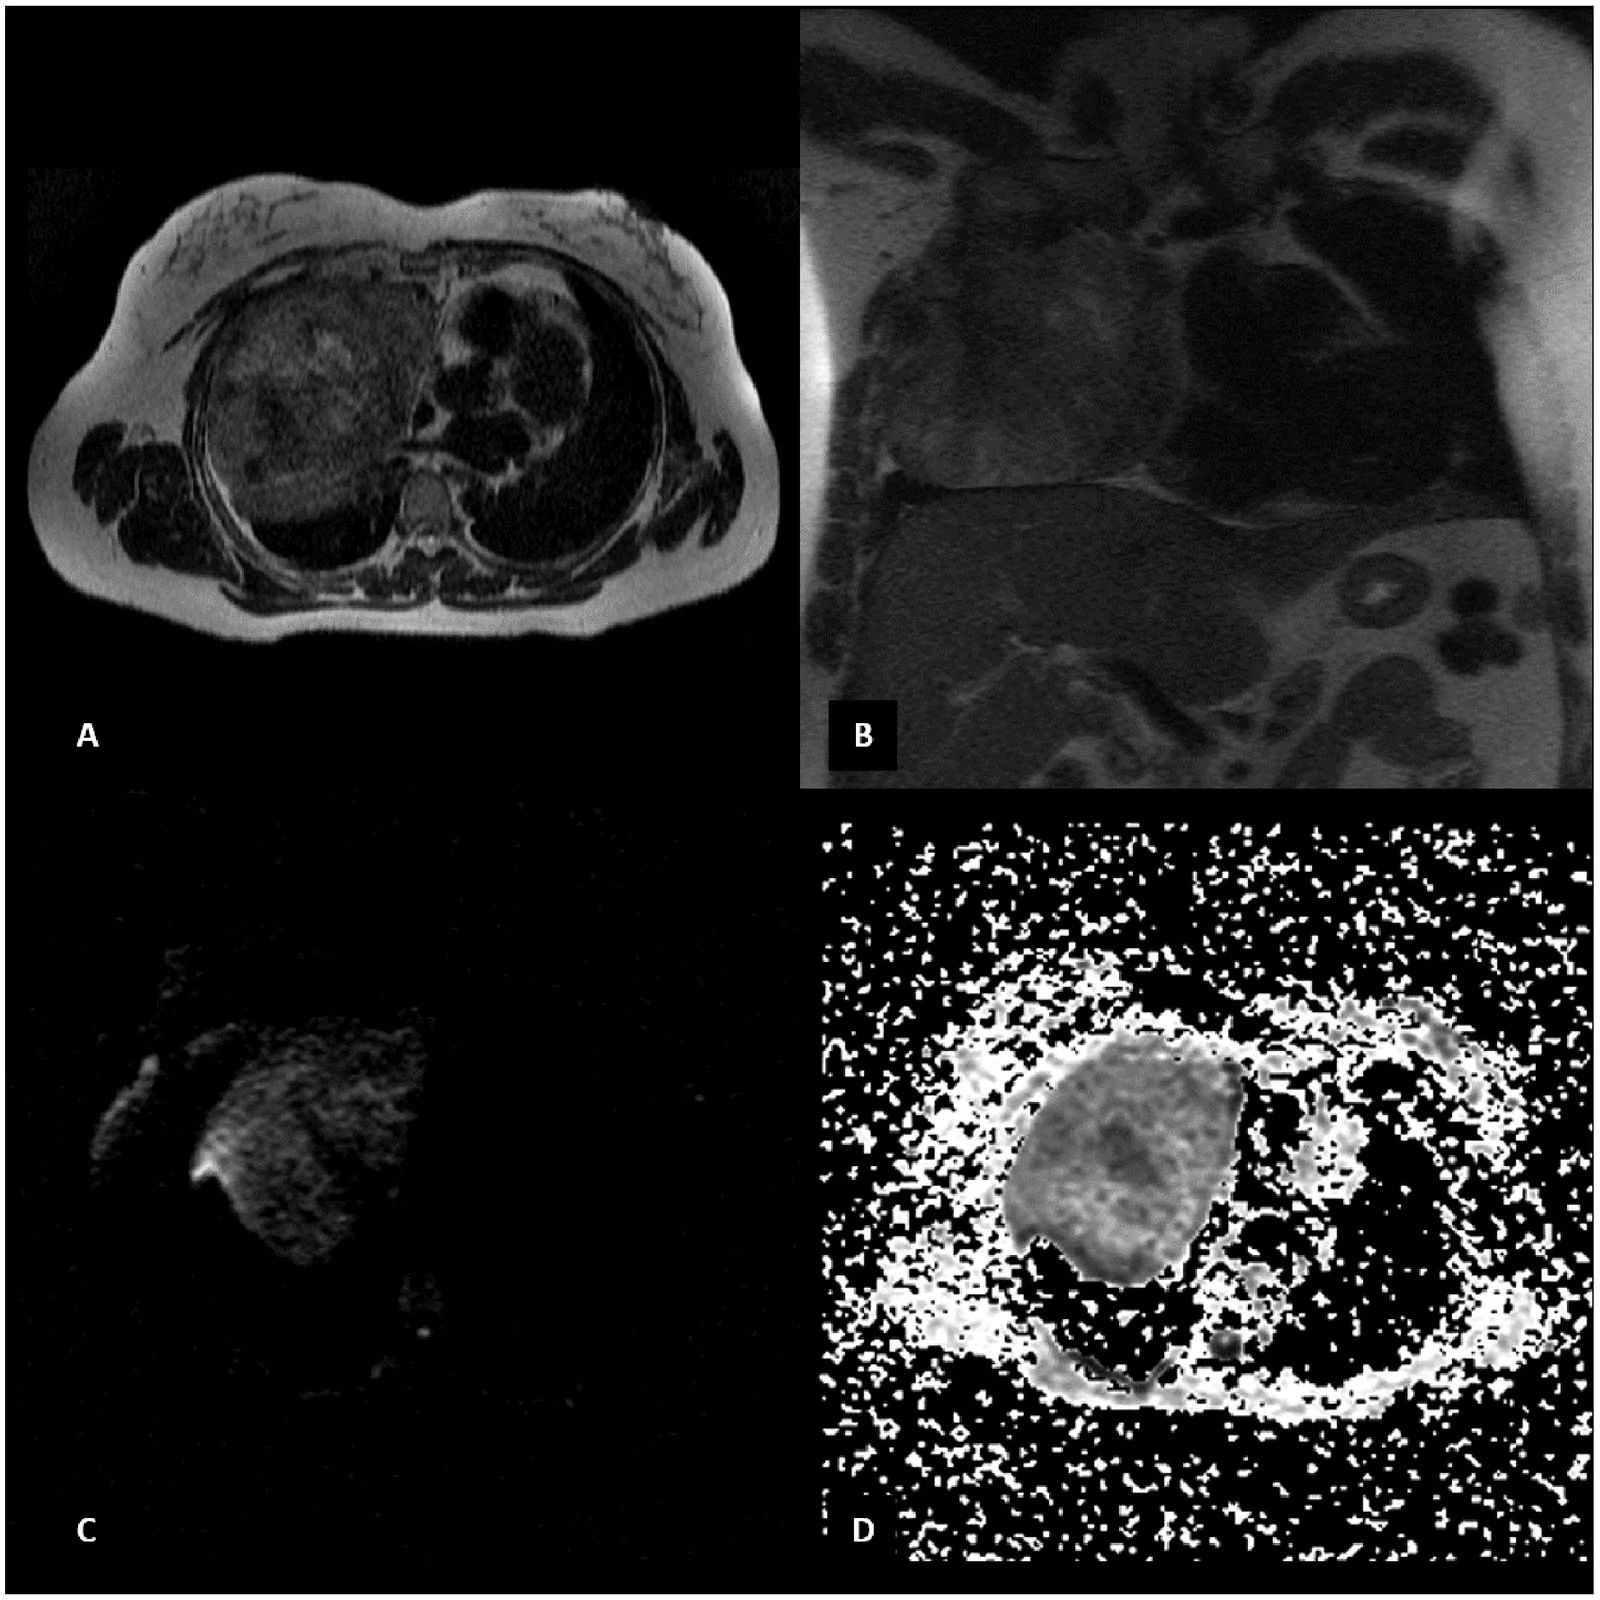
Preoperative MRI obtained 4 days after admission. (A and B) T2-weighted single-shot fast spin-echo (SSFSE) images show a pleural-based mass with heterogeneous, predominantly intermediate signal intensity. (C) Diffuse-weighted imaging (DWI) at b = 800 s/mm² demonstrates heterogeneous restricted diffusion. (D) The corresponding apparent diffusion coefficient (ADC) map confirms diffusion restriction.
